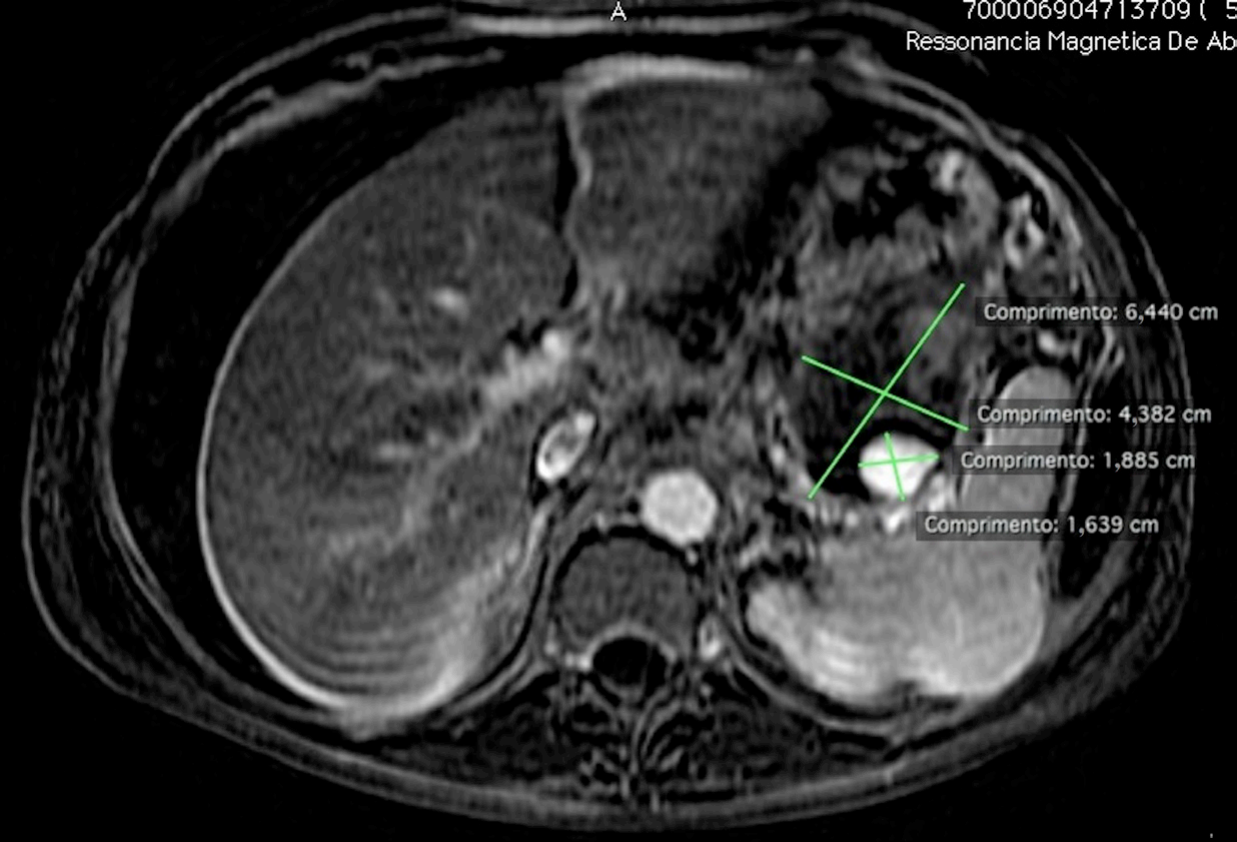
Magnetic resonance image of the abdomen in T1 with fat saturation after subtraction showing a pseudoaneurysm of the splenic artery close to the splenic hilum, surrounded by an oval-shaped mass with thick/hematic content.
